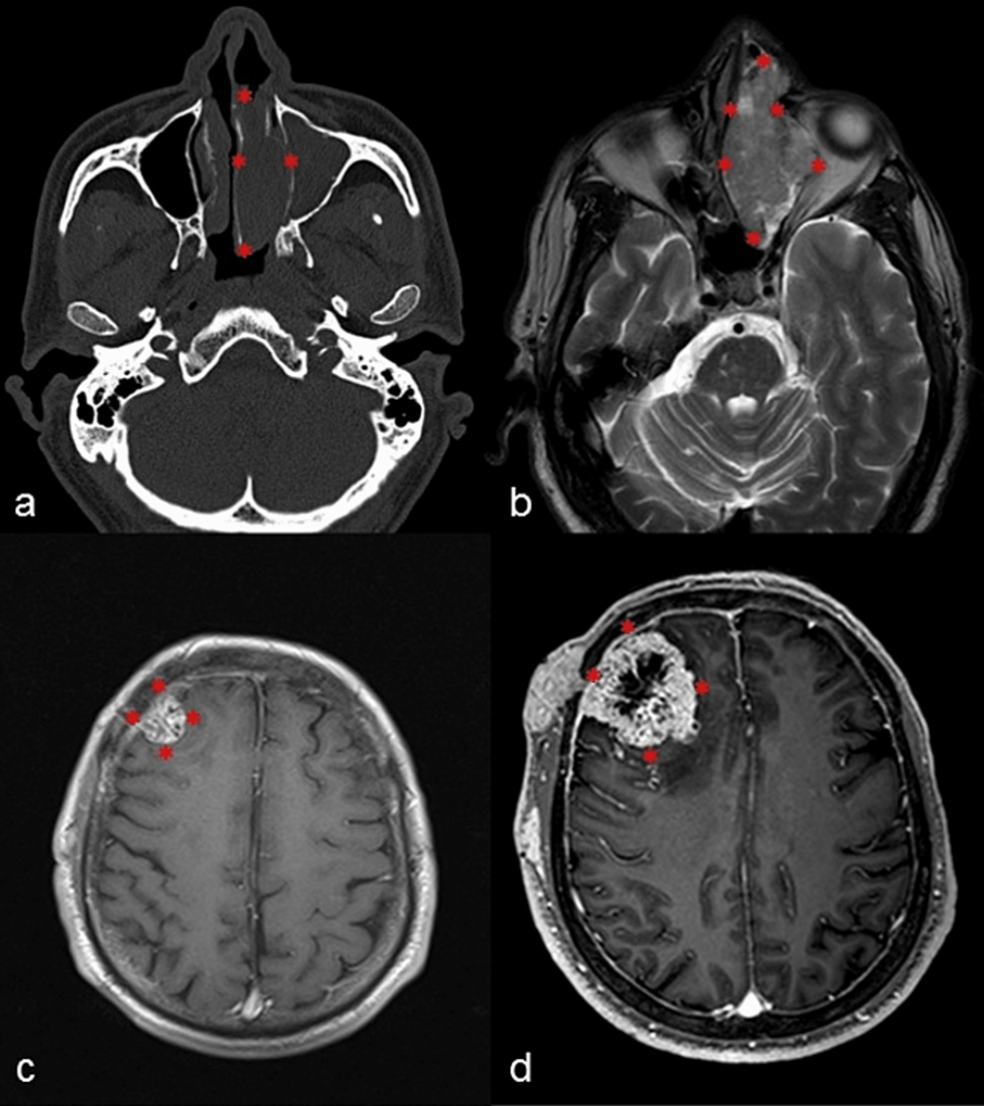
Images of the HMSC a) CT scan of a single axial skull with tumor lesion (asterisk) in an expansive hypodense nasal cavity with remodeling of the medial wall of the maxillary antrum, conditioning obstruction of the maxillary sinus, anterior and posterior ethmoid cells; b) T2 MRI amorphous tumor lesion in nasal cavity (asterisk) with extension to anterior and posterior ethmoid cells with infiltration to the medial wall of the orbit  c) Axial T1 MRI with contrast with extraaxial heterogeneous intracranial metastatic lesion (asterisk) with extension to meninges and soft tissues; d) T1 MRI with axial contrast five months later with disease progression (asterisk).
